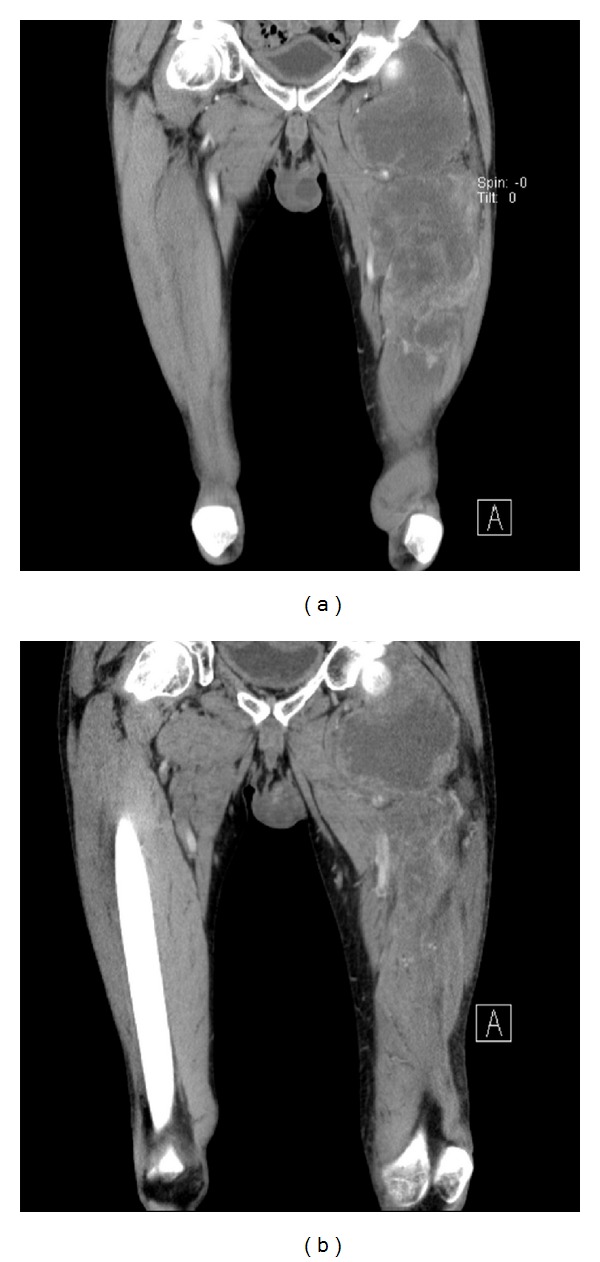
CT scan, (a) before the start of CTx and (b) after 2 cycles of CTx.
